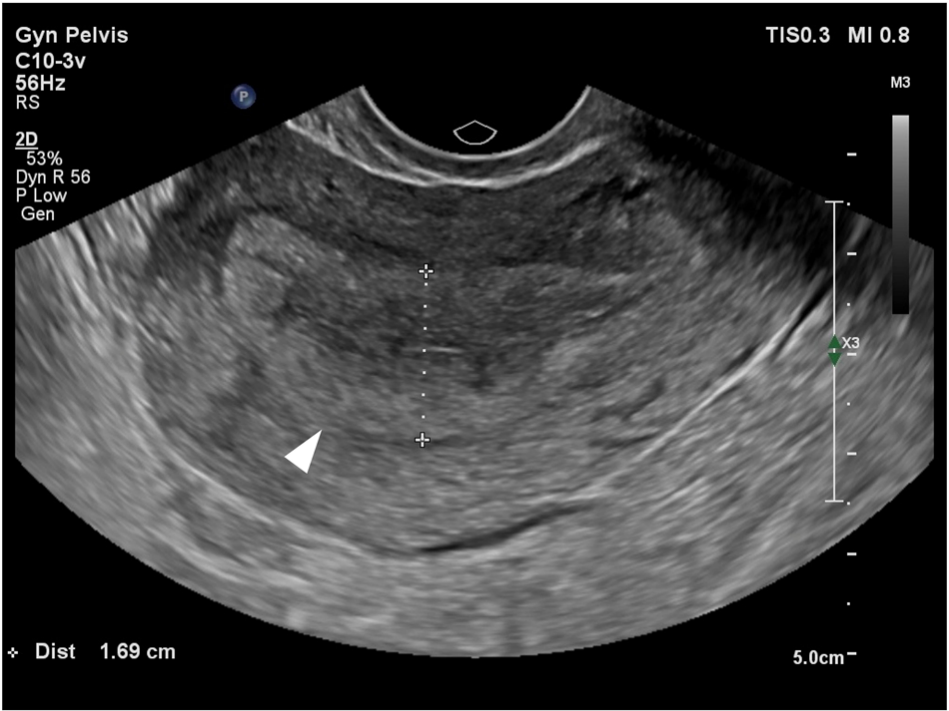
An example of an endometrial thickness measurement via transvaginal ultrasound. The uterine sagittal view shows the endometrial echo (arrowheads) extending from the fundus to the cervix. A dashed caliper line is placed perpendicular to the endometrial midline, with endpoints aligned at the outer borders of the endometrium, which yields a thickness of 1.69 cm
